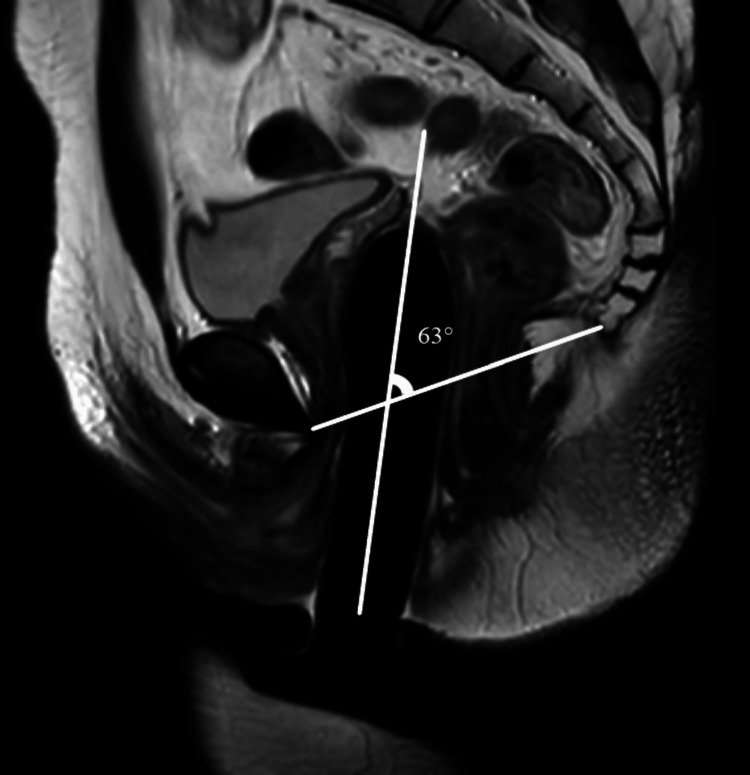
T2W HR sagittal image of a non-defaulter patient Original MRI image of a patient who underwent the study.
